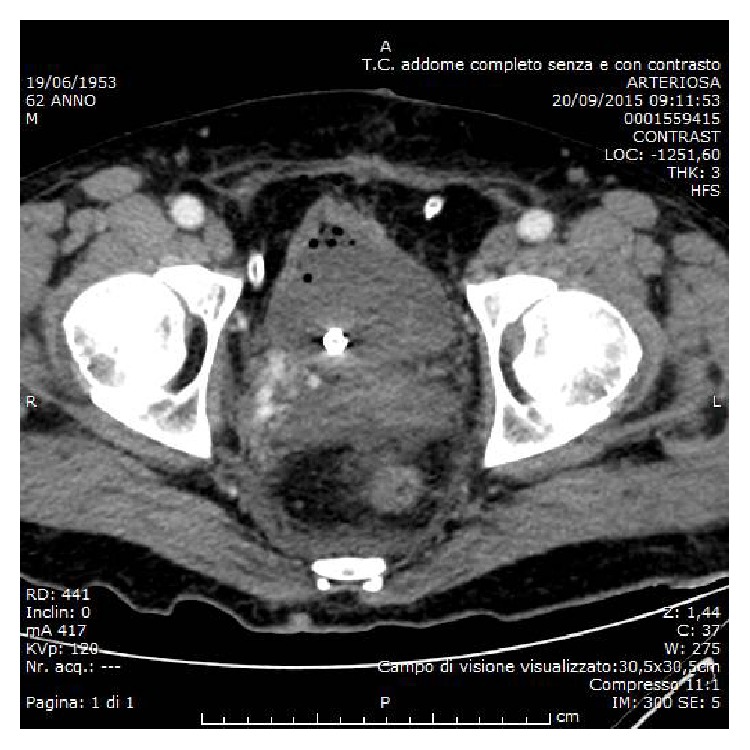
Contrast-enhanced CT scan, angiographic phase: abnormal extravasation of contrast medium in the soft tissue near bladder wall.
